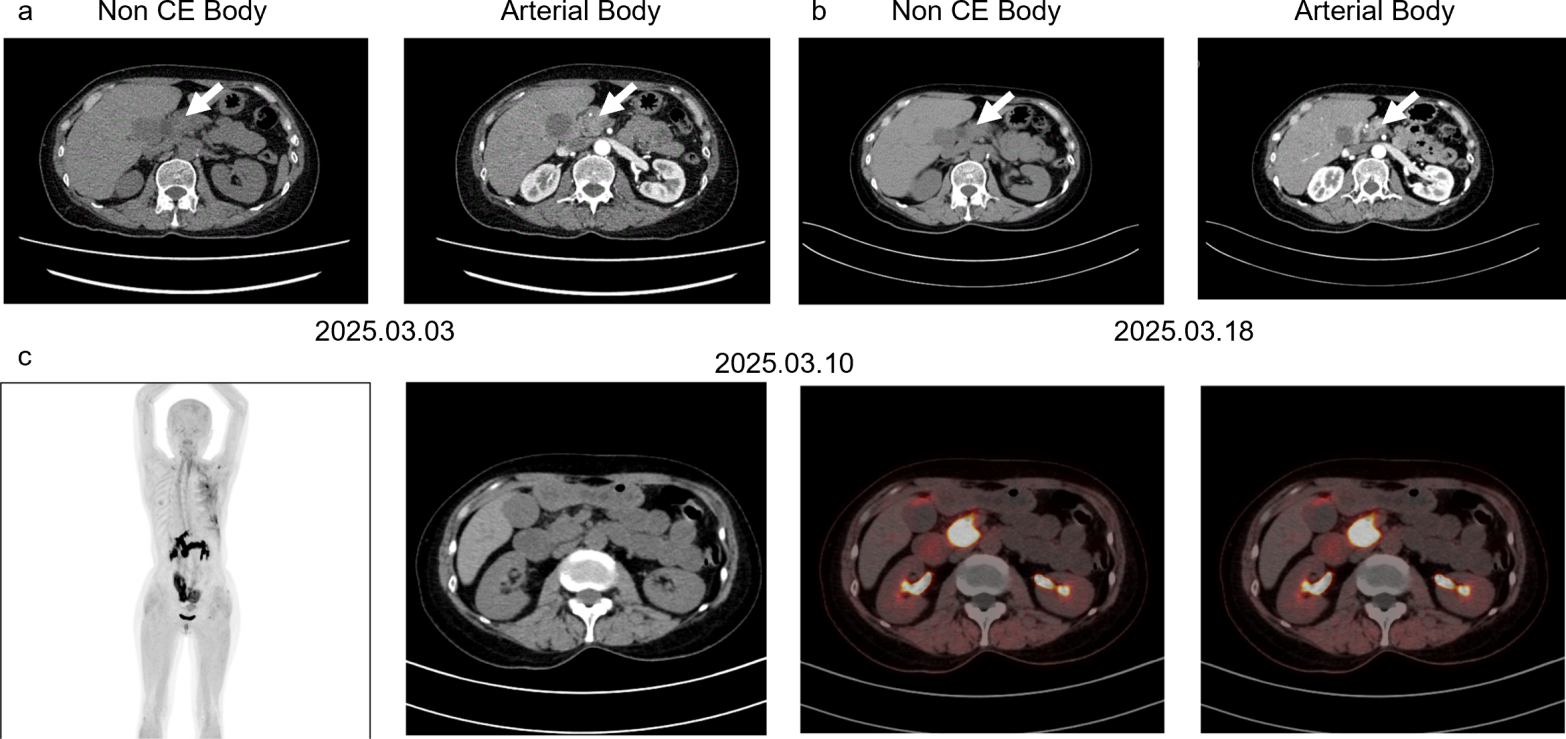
Radiological results for this patient. (a, b) are two CT results in March 2025. On March 1, 2025, an ill-defined, swollen mass was identified in the pancreatic head without appreciable delayed-phase enhancement. On March 18, 2025, the lesion was more clearly delineated, with reduced surrounding swelling and progressive delayed-phase enhancement, suggesting a substantial fibrous component and suspicious malignancy. (c) is the FAPI PET-CT result.
